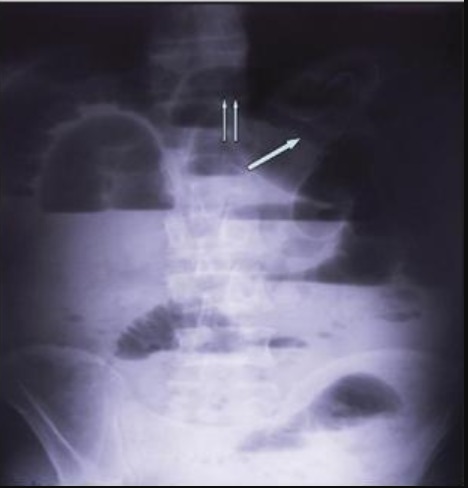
Radiographie d'abdomen sans préparation de face debout montrant de multiples niveaux hydro-aériques de type grêlique dont certains siègent au niveau de l'hypochondre droit. Présence d'une anse plate (flèche simple) contenant de l'air en continuité avec une anse distendue avec une zone de transition (flèche double) se projetant en regard de l'aire hépatique
